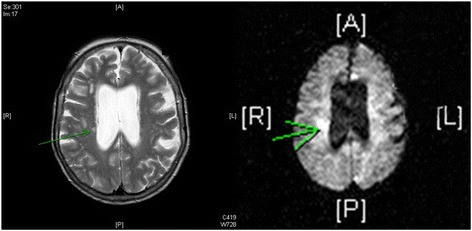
Magnetic resonance imaging scan showing the suspected infarction in the right corona radiata, *green* arrows (*left*: T2-weighted image, *right*: diffusion-weighted image)
